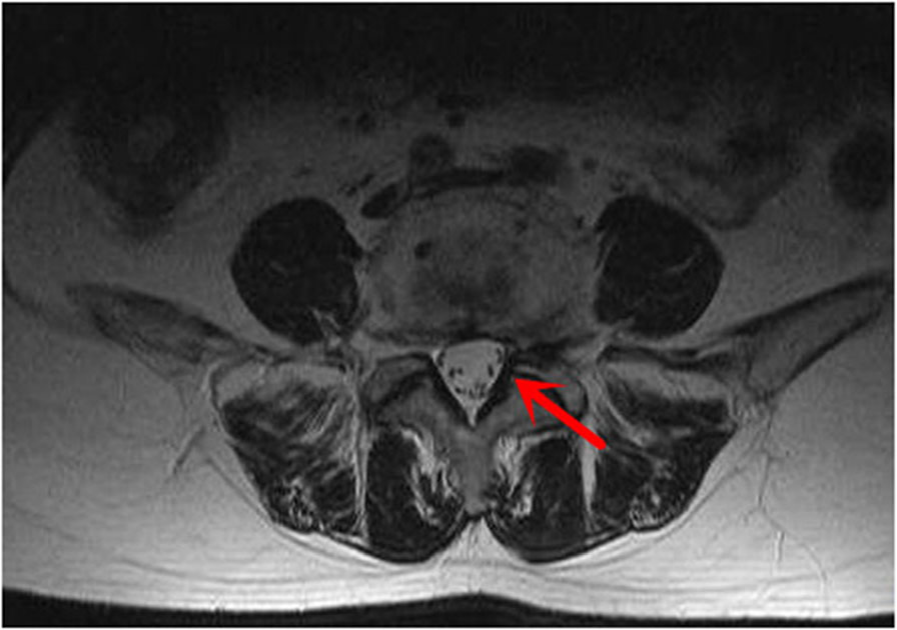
After postoperative 12 months, an MRI scan showed a complete absence of the gas discal cyst at the site of treatment (red arrow)
